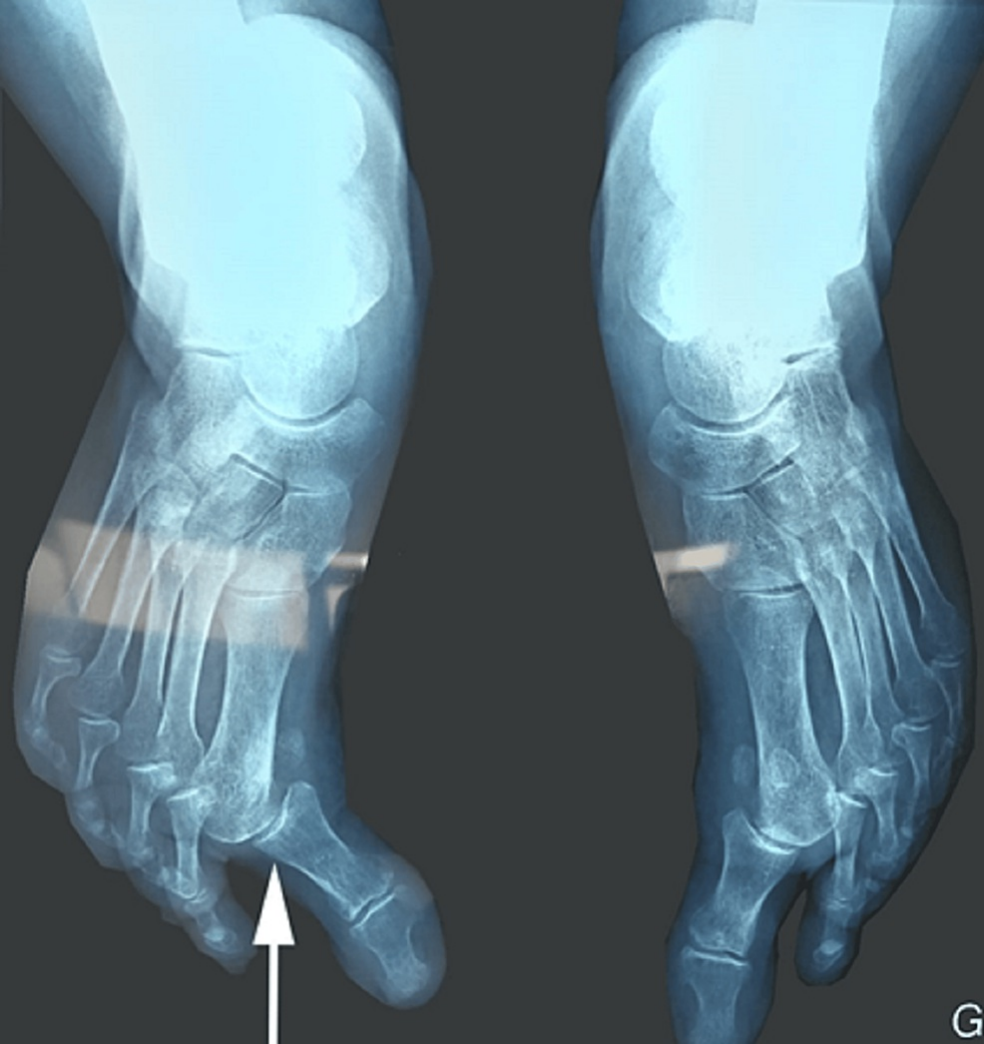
An anteroposterior standard foot radiograph showing bilateral dislocation of the first MTP joint more significant on the right (white arrow) MTP, metatarsophalangeal
